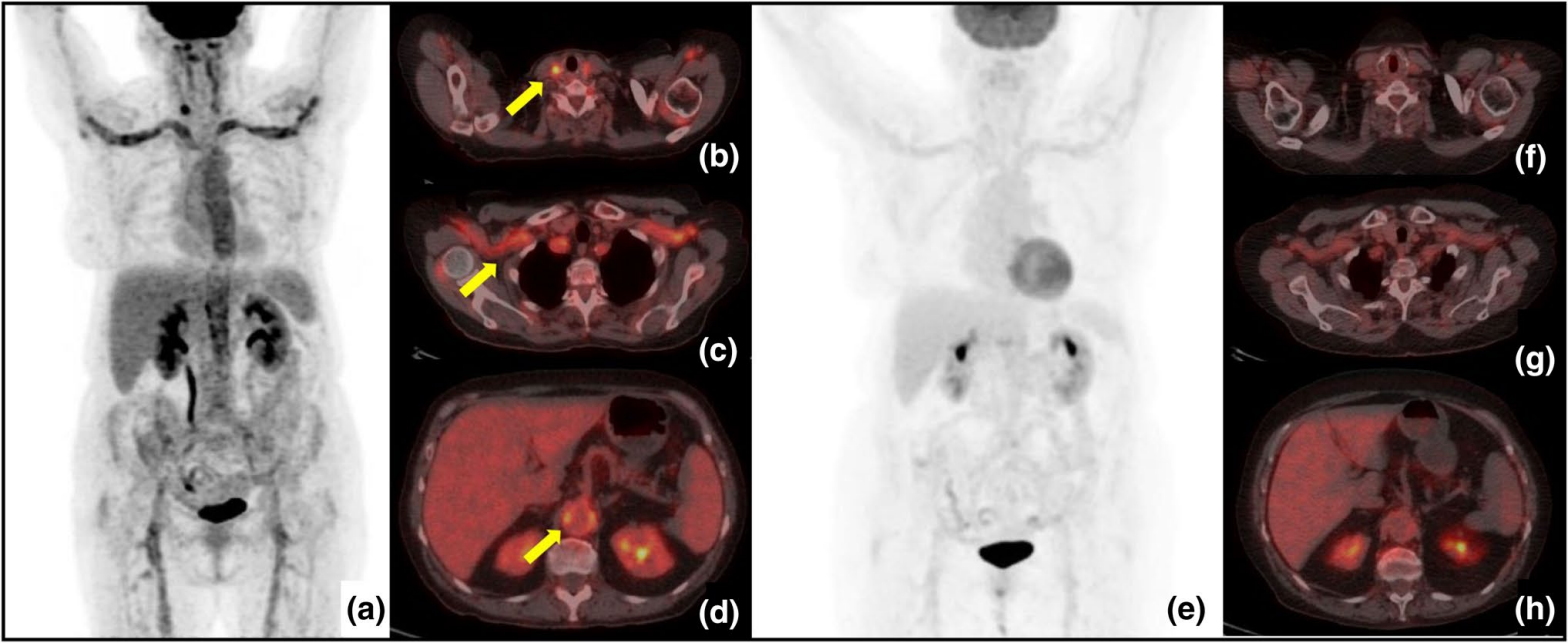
^18^F-FDG PET/CT in a 42-year-old female patient with type V Takayasu arteritis who complained of fatigue, weight loss, fever, and severe headache. Maximum intensity projection (MIP) (a) and axial fused images showed increased ^18^F-FDG uptake (yellow arrows) in the aorta and its main branches, particularly in carotid (b) and subclavian (c) arteries and in the distal abdominal aortic wall (d). After 6 months of therapy, follow-up PET/CT images show clearly decreased uptake in the large arteries (E–H)
